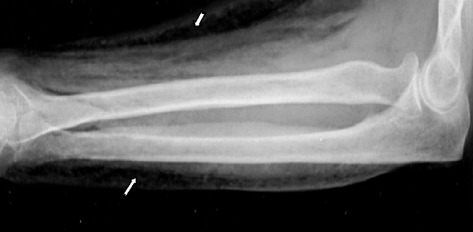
X-ray profile of her right arm showing subcutaneous emphysema (arrows)
